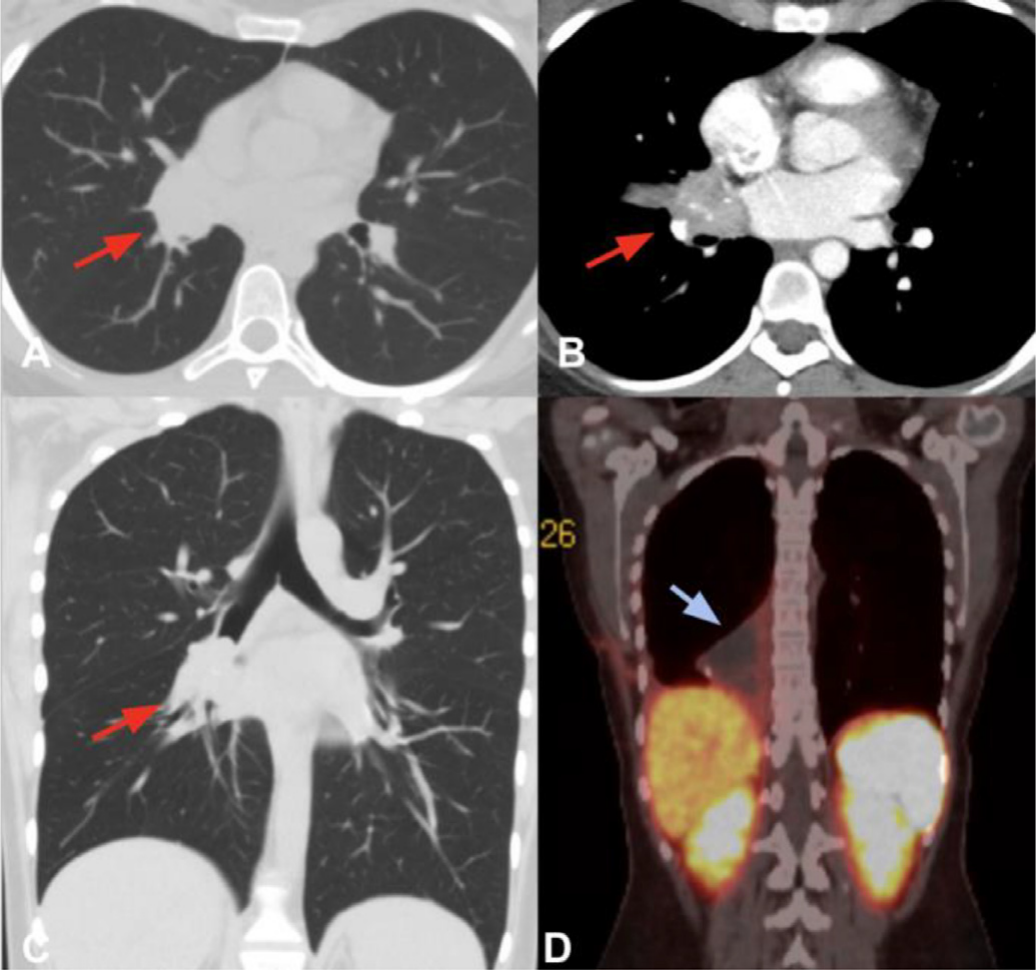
Computed tomography of thorax in axial (A) and coronal (C) sections in lung window without contrast shows a parahilar mass with regular borders and in an axial section with contrast in arterial phase (B) shows calcifications in its interior involving the right bronchus intermedius (red arrow). Postsurgical Ga68-Dotanoc PET/CT (D) without evidence of somatostatin receptor overexposure lesions shows nodule (white arrow) with moderate somatostatin receptor expression (Krenning 2), located in the retroareolar region of the right breast of 28 mm (SUVmax 5.5).
